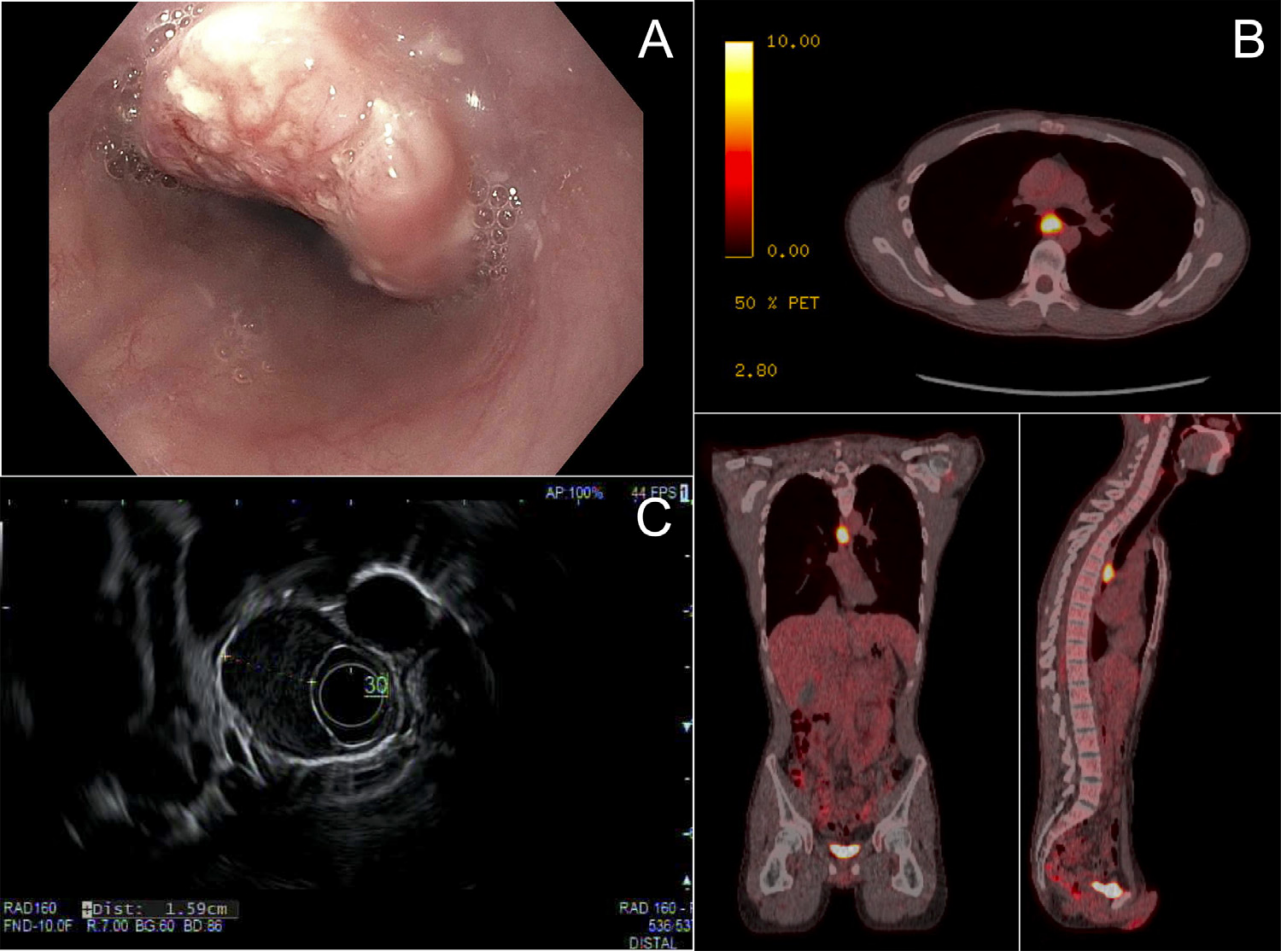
Staging at time of diagnosis. (A) Esophagogastroduodenoscopy revealing a friable, ulcerating nonobstructive mass in the proximal esophagus extending 25 cm to 28 cm from the incisors, obstructing about 50% of the esophageal lumen. (B) Positron emission tomography/computed tomography showing fluorodeoxyglucose-avid mass in the midesophagus (SUV max = 15.6). No fluorodeoxyglucose-avid lymph nodes or distant metastases were visualized. (C) Endoscopic ultrasound of midesophageal mass measuring 16 mm in thickness invading into the muscularis propria.
